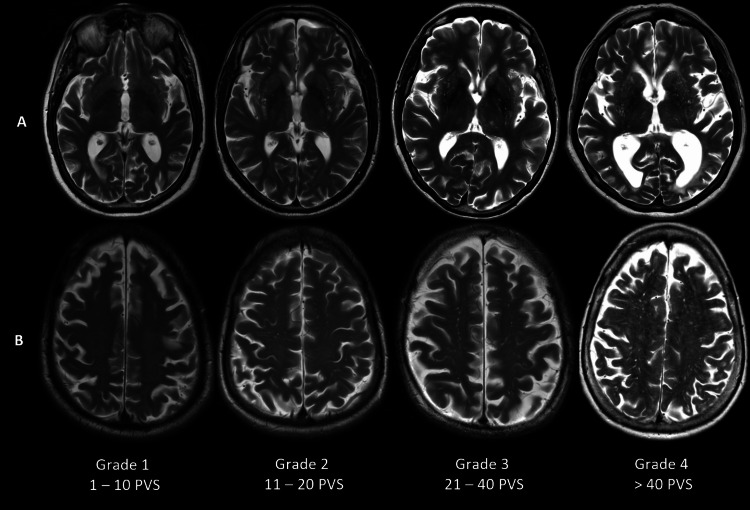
Axial T2-weighted images showing dilated perivascular spaces (PVS) in the basal ganglia (a) and centrum semiovale (b) from Grade 1 to Grade 4.
